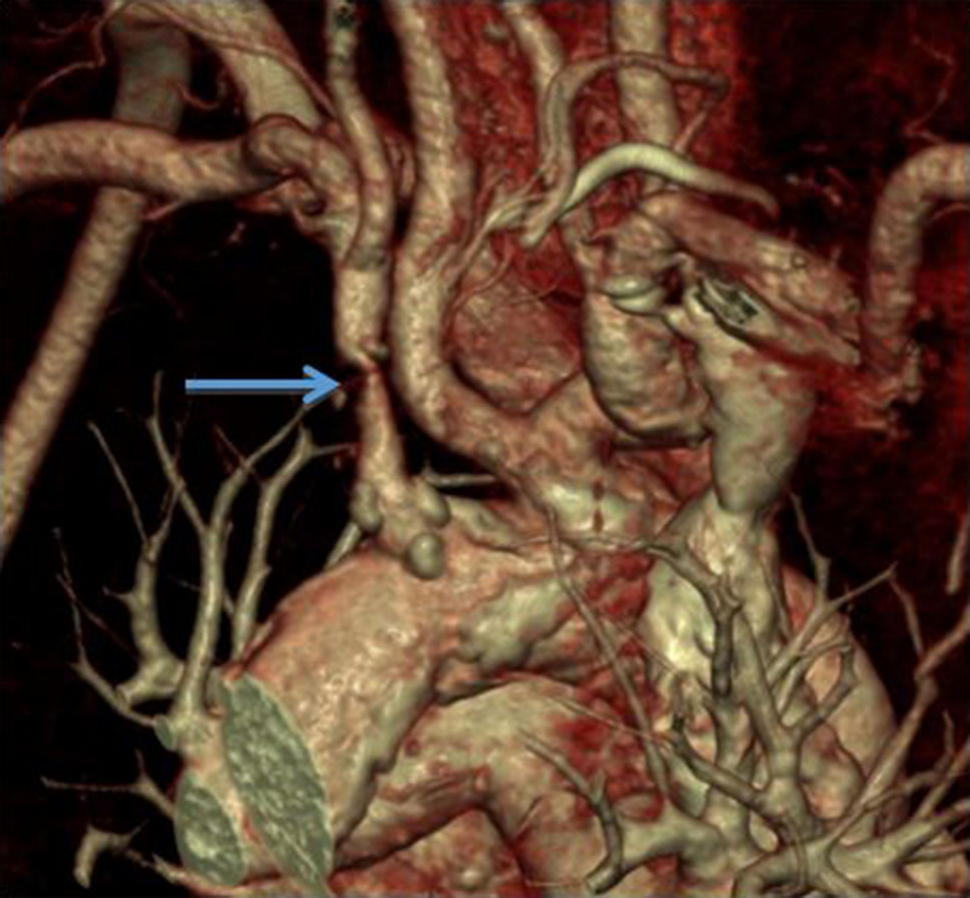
3D reconstruction CT scan of an aortic arch in a patient with a poorly maturing AV fistula. A proximal subclavian artery stenosis was identified (arrow) and subsequently treated with an endovascular stent.
